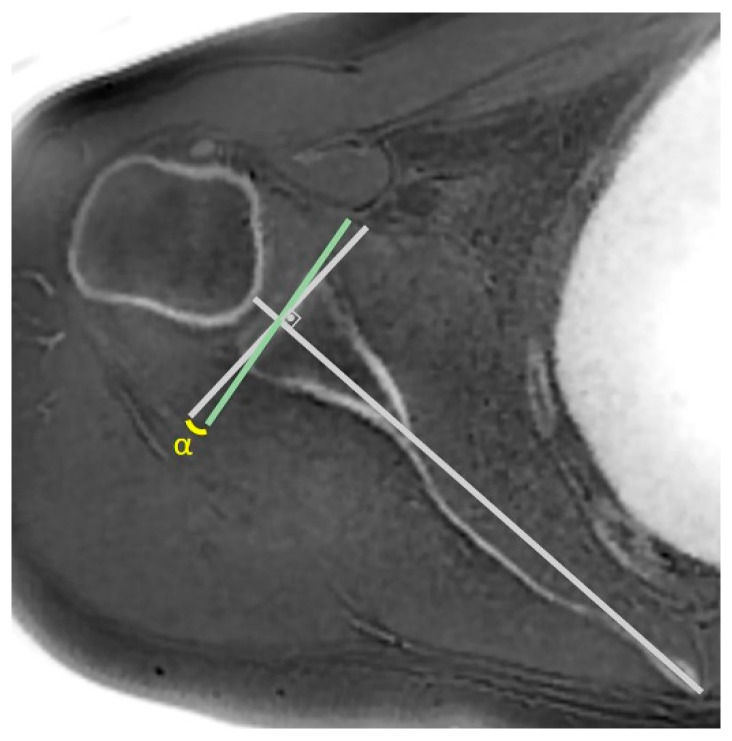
Glenoid version was measured on 2D ‘true scapular plane’ reformats generated from the 3D-reconstructed ZTE MRI images using the Friedman method. A line was drawn from the medial border of the scapula (medial tip of the scapular spine) to the midpoint of the glenoid cavity (the Friedman line). The angle between a line perpendicular to the Friedman line and a line connecting the anterior and posterior rims of the glenoid was defined as the glenoid version.
